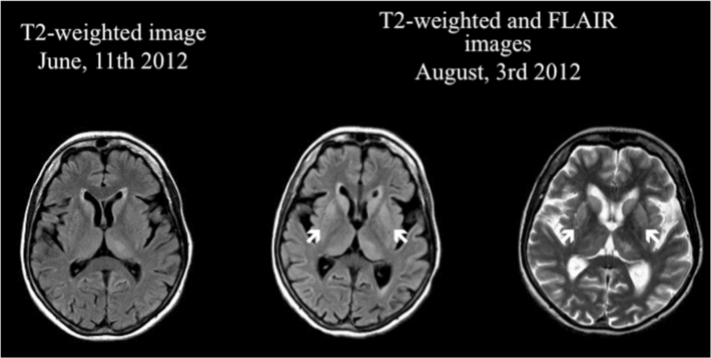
MRI formation of symmetrical hyperintensities in the putamen and caudate head within two months seen on T2-weighted and FLAIR images.
